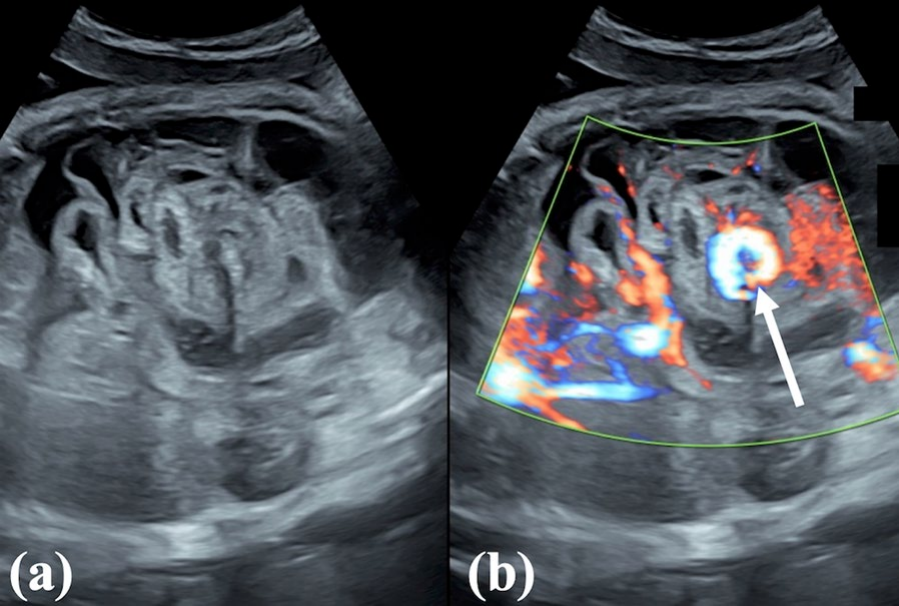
Fetal ultrasound: a fetal bowel is dilated with some ascites. b Vessels of the bowel are twisted (whirlpool sign, arrow)
